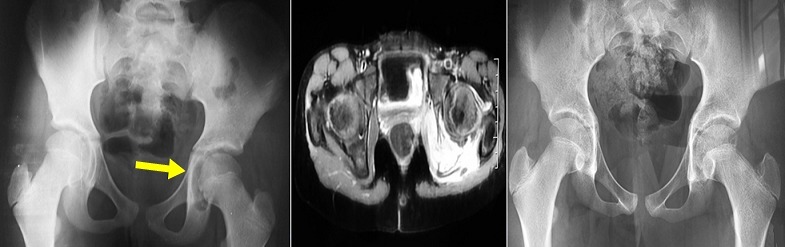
A) pelvic X-ray showed an osteolysis of the roof of the left acetabulum with regular contour; B) MRI of the hip joint showed tissue thinning and damage of the roof of the left acetabulum; C) pelvic X-ray after 1 year showed a filling of the gap
